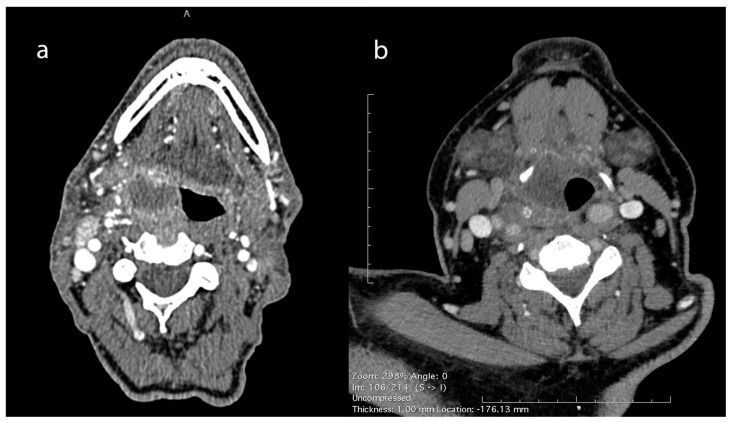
CT scan with contrast of pharyngeal mucosal space abscesses (PMAs). (a) Right-side PMA protruding into the pharyngeal lumen, located towards the lateral pharyngeal wall, just under the pharyngeal mucosa and medial to the pharyngeal constrictors, away from the ipsilateral carotid artery and the internal jugular vein. (b) Right-side PMA protruding into the pharyngeal lumen and passing medially to the hyoid bone, away from the ipsilateral carotid artery and the internal jugular vein.
